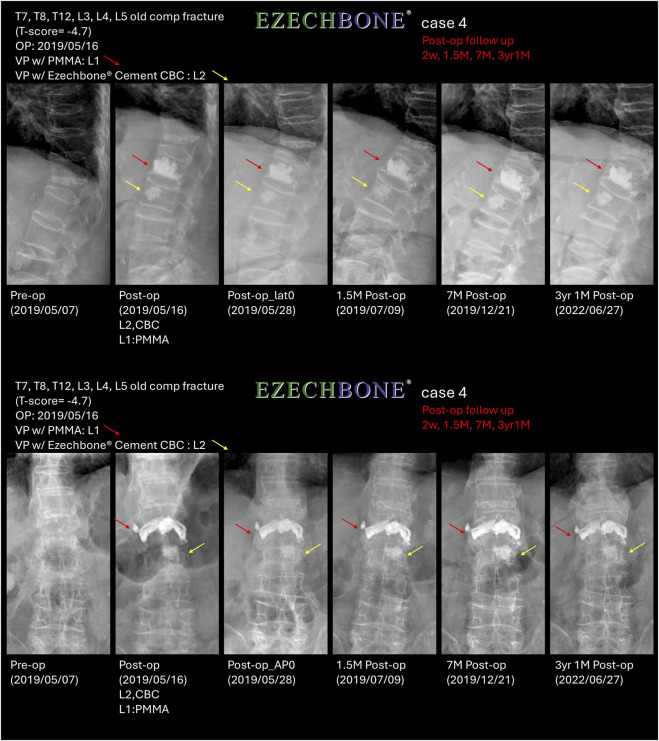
The preoperative and a series of follow-up radiographs in case 4.
